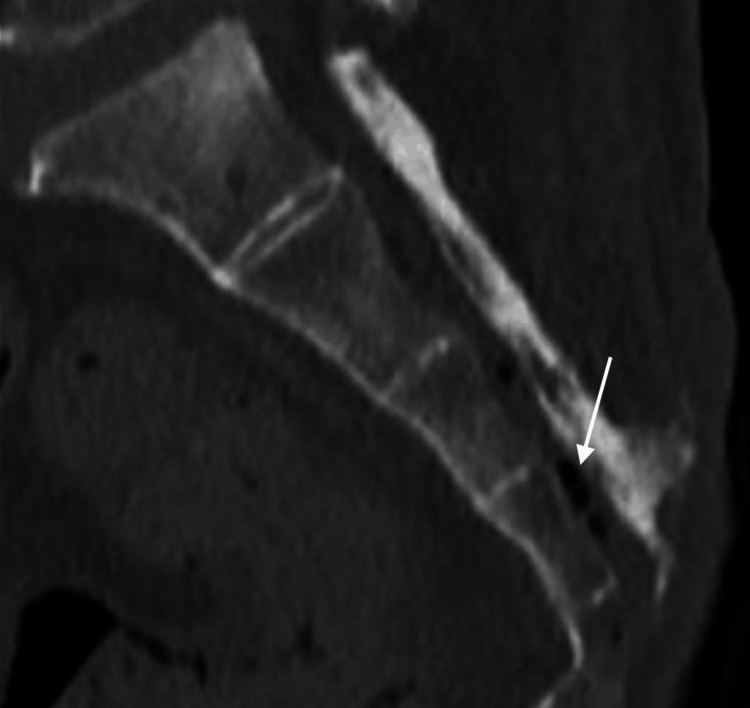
CT scan of distal sacrum and coccyx CT scan of sacrum and coccyx showing osteomyelitis and erosion with gas formation in spinal canal suggesting progressive worsening of condition with introduction of infection into spinal canal (white arrow).
